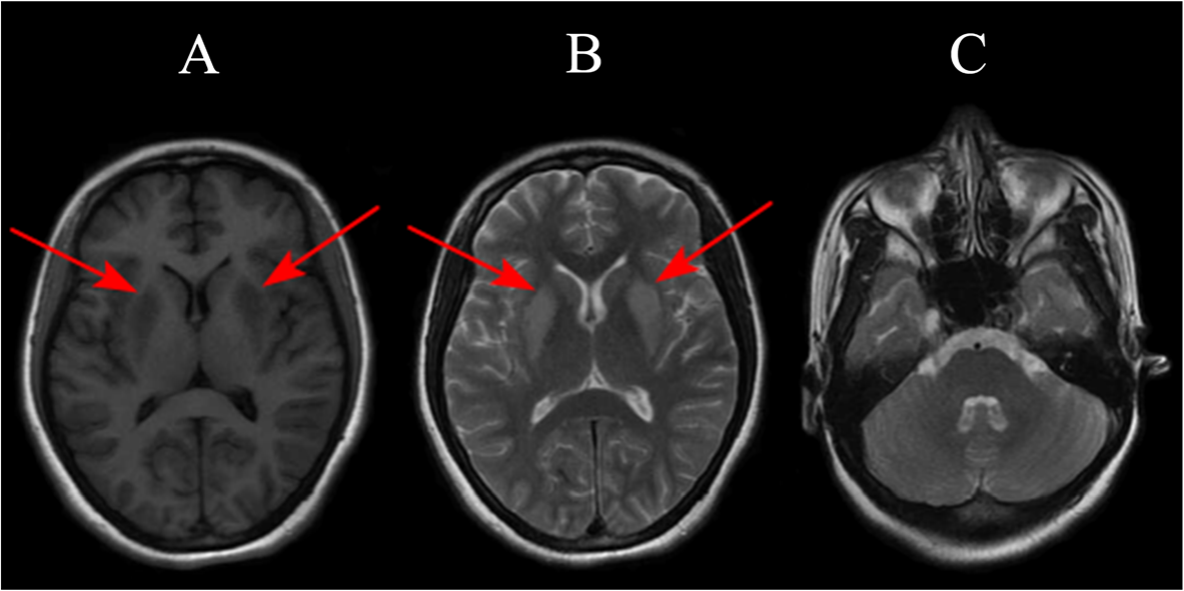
Brain magnetic resonance imaging. A, axial T1-weighted MRI showing low signal intensity of bilateral symmetric basal ganglia; B, axial T2-weighted MRI showing high signal intensity of bilateral symmetric basal ganglia; C, axial T2-weighted MRI showing no lesion in the pons (the red arrows pointing out the lesions).
